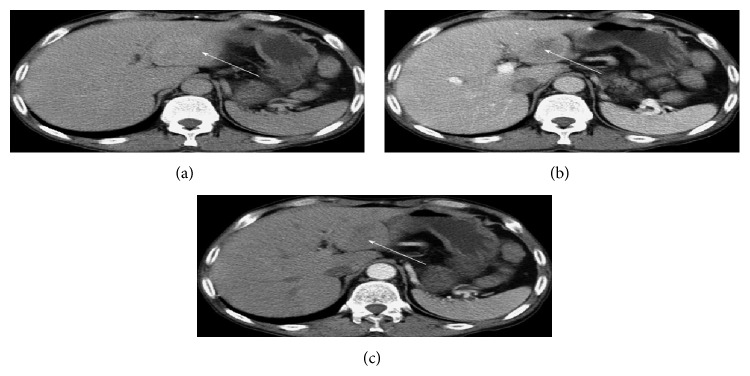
CT Abdomen with a mass in segment III of the liver in the hepatic equilibrium (a), portal venous (b), and hepatic arterial phase (c). Reprinted from [2] with the permission of the Director, Editorial Office of the World Journal of Gastroenterology.
